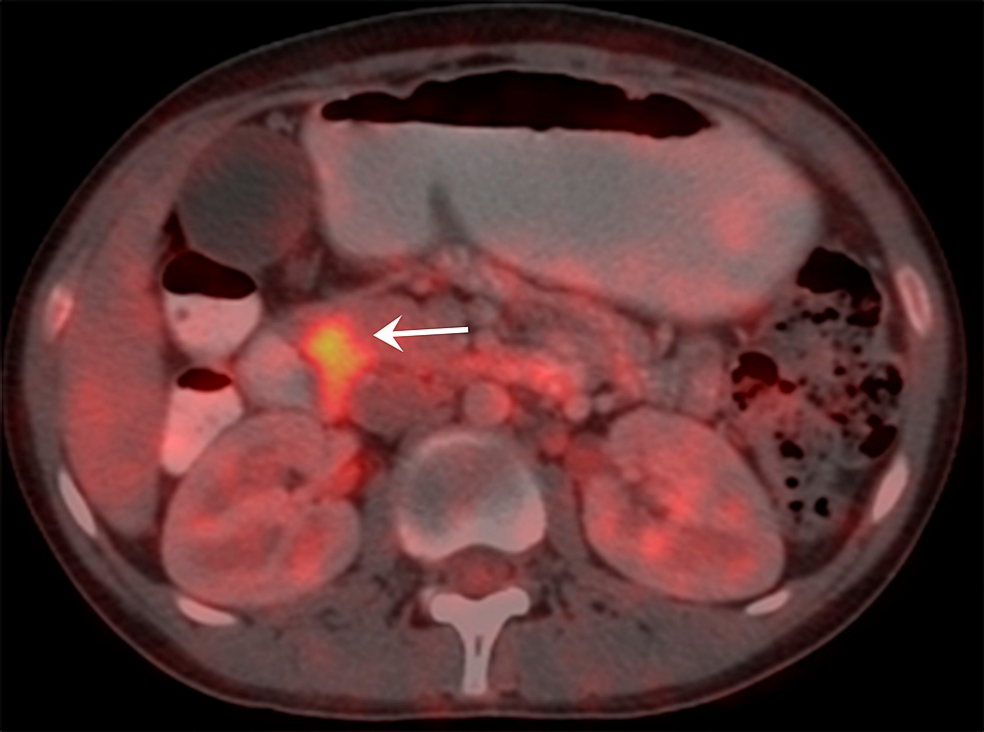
18F-FDG PET-CT showing FDG avid soft tissue periampullary thickening measuring ~2.7 × 1.2 cm with a maximum standard unit value (SUVmax) of 6.9 extending into the D2–D3 junction with ill-defined fat planes with pancreas medially (arrow).
